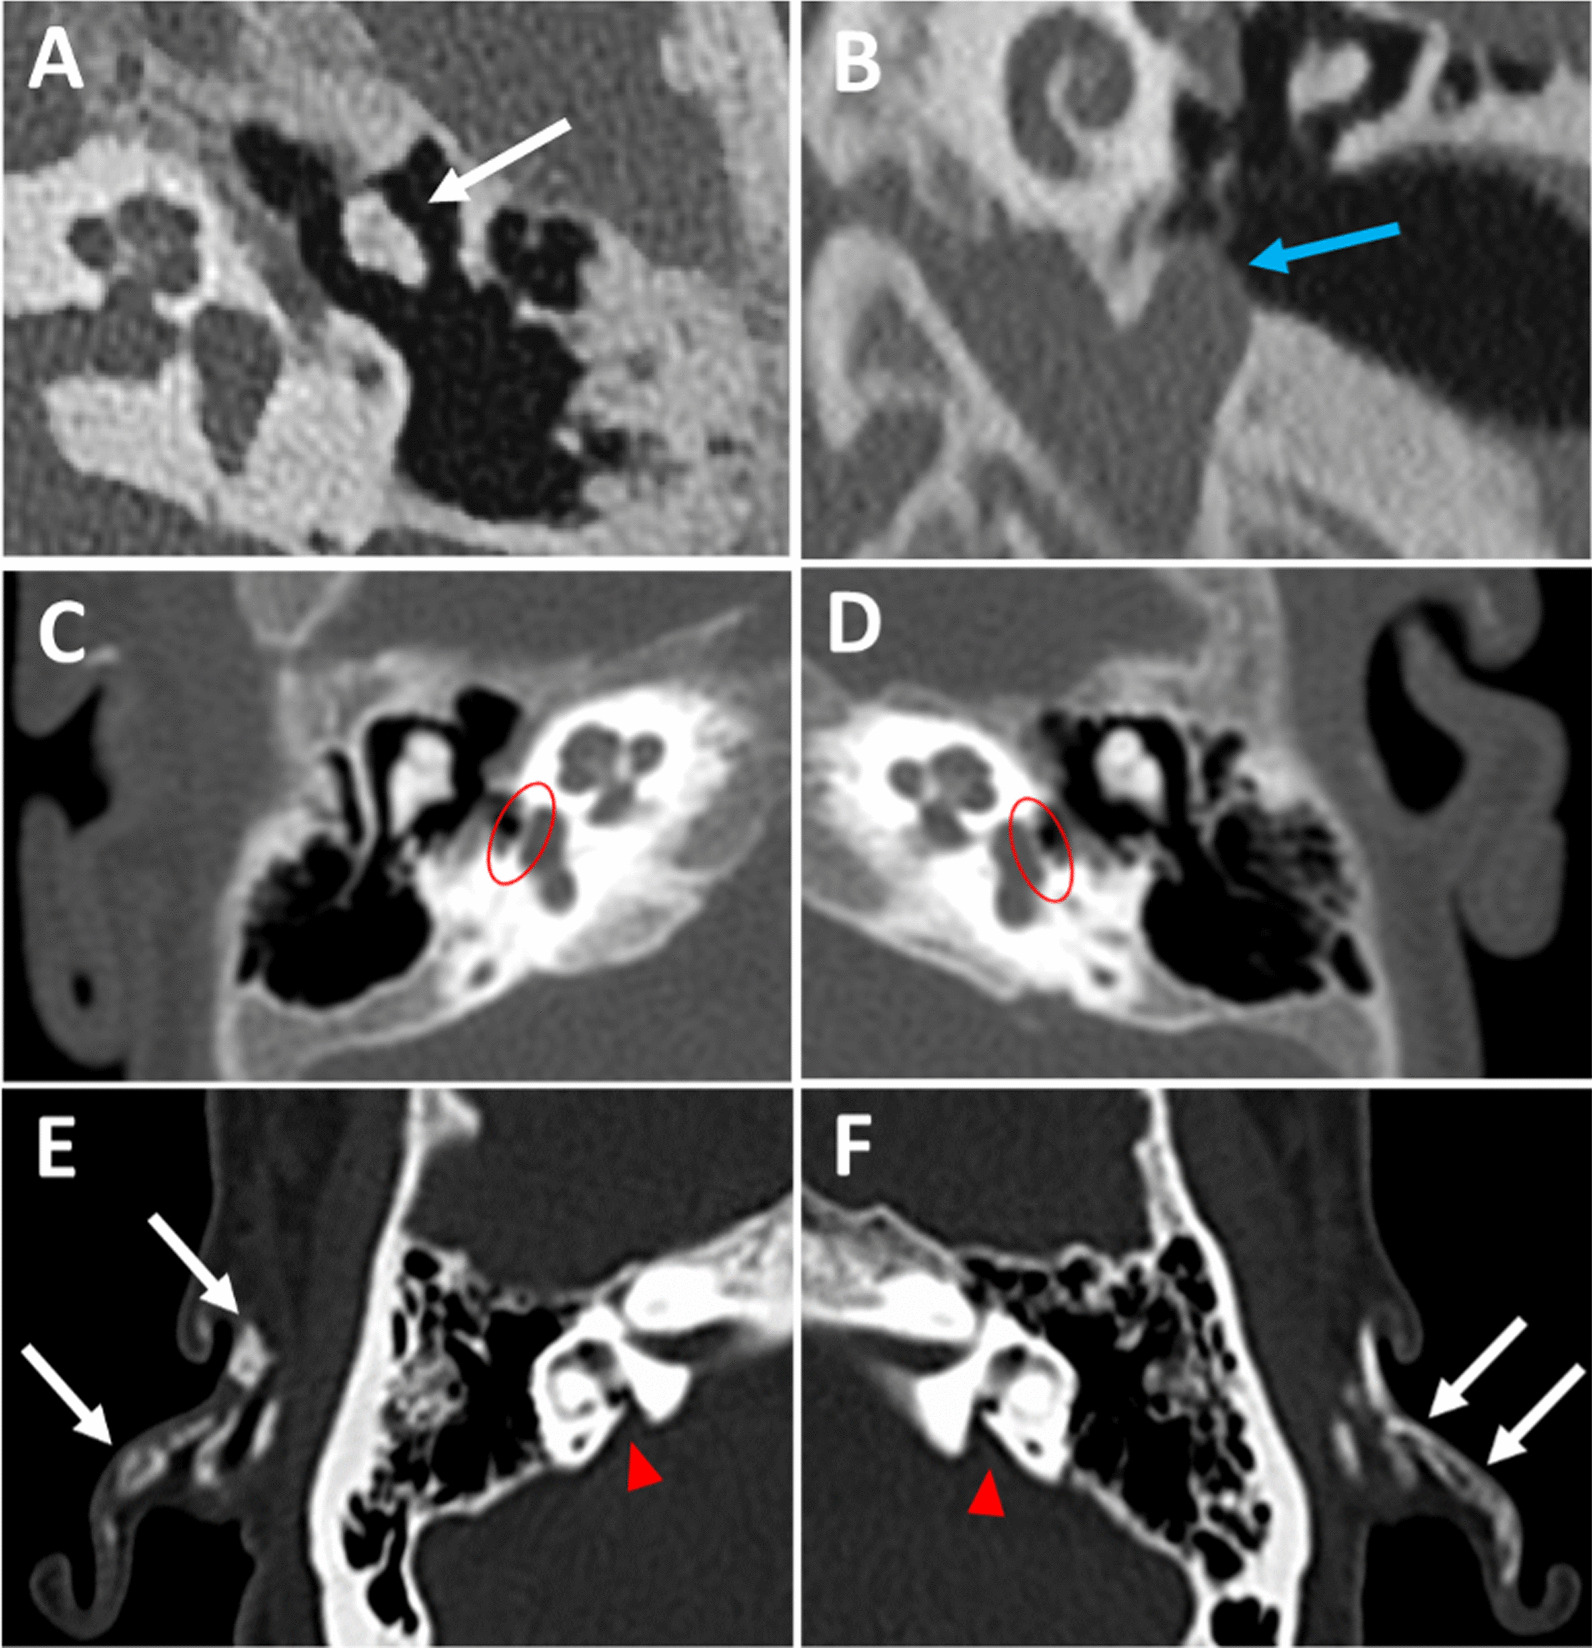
Temporal bone CT images of GACI patients. Abnormal findings (A–D) in the 25-year-old GACI patient, including an axial view of (A) an abnormal malleus and incus in the left ear (white arrow), a coronal view of a (B) left-sided high-riding jugular bulb with diverticulum (blue arrow), and axial views of (C) right and (D) left calcified stapes footplate (encircled). Abnormal findings (E–F) in the 35-year-old GACI patient were bilateral auricular calcifications (white arrows) and bilateral enlarged vestibular aqueduct (arrowheads)
